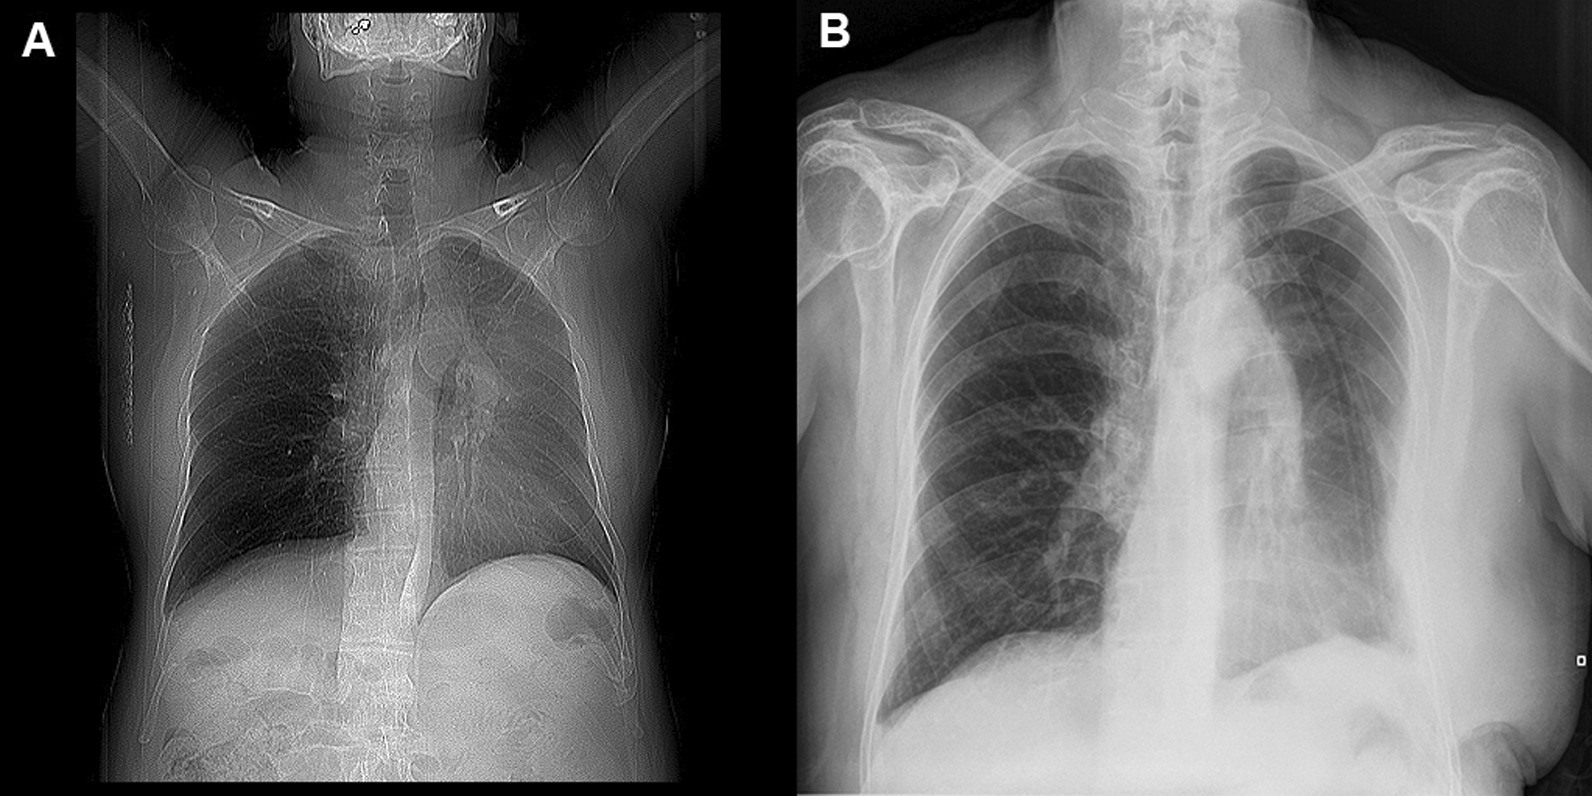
A Preoperative X-ray of the chest showing increased density of the left lung field. B Postoperative X-ray of the chest showing increased density in the lower zone of the left lung field resulting from left upper lobectomy
